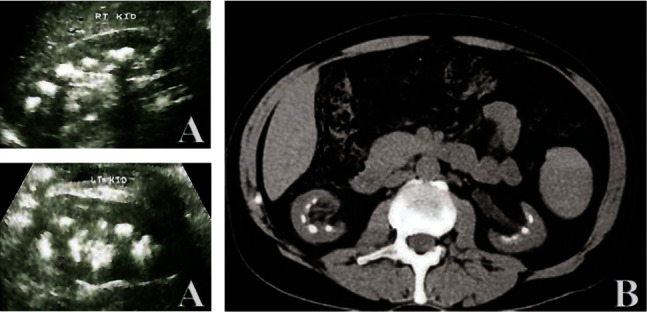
Ultrasound showing a small right kidney with nephrocalcinosis and calcified papilla (a) that was confirmed by CT scan (b).
